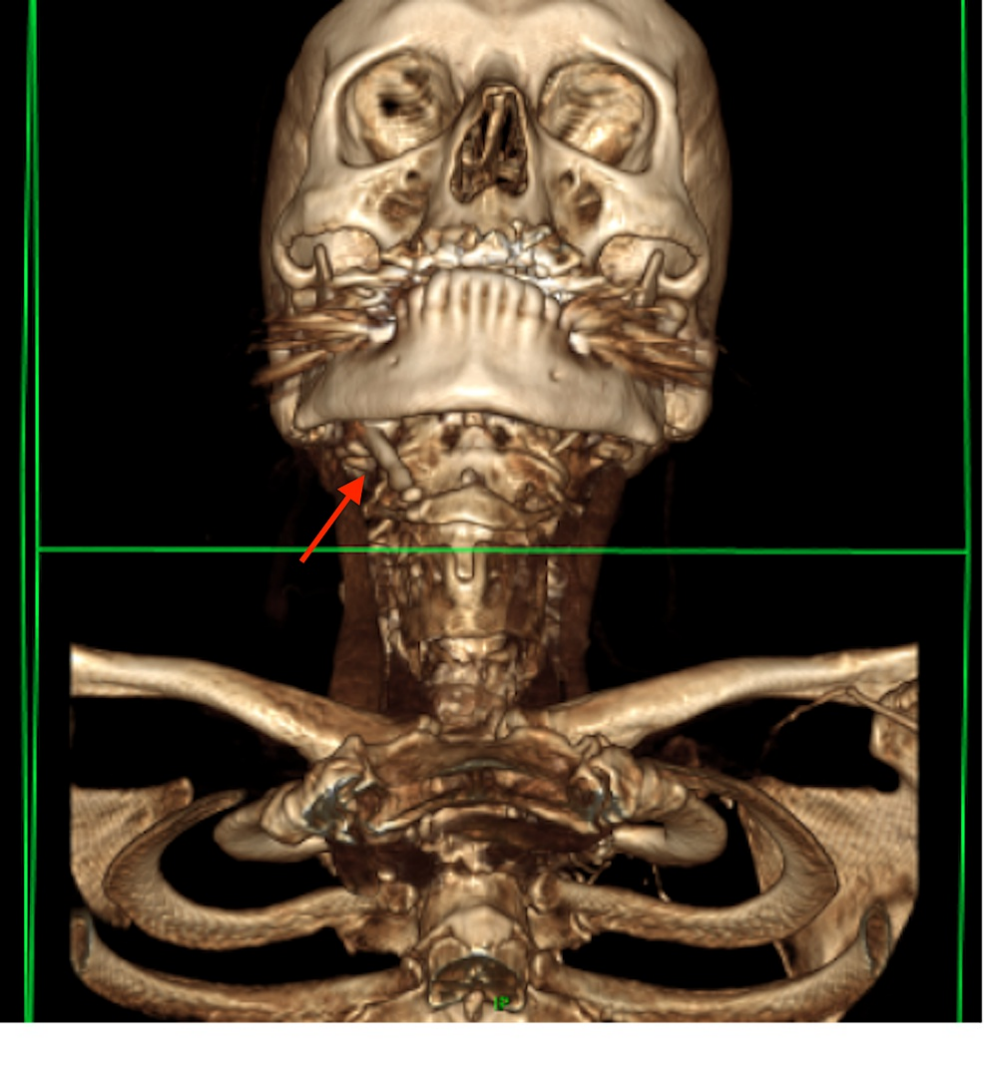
Three-dimensional CT scan of the head and neck showing enlarged, thickened right styloid process with complete ossification of the stylohyoid ligament, and significant bony overgrowth of the pseudoarticulation between the tip of the styloid process and the ossified ligament.
